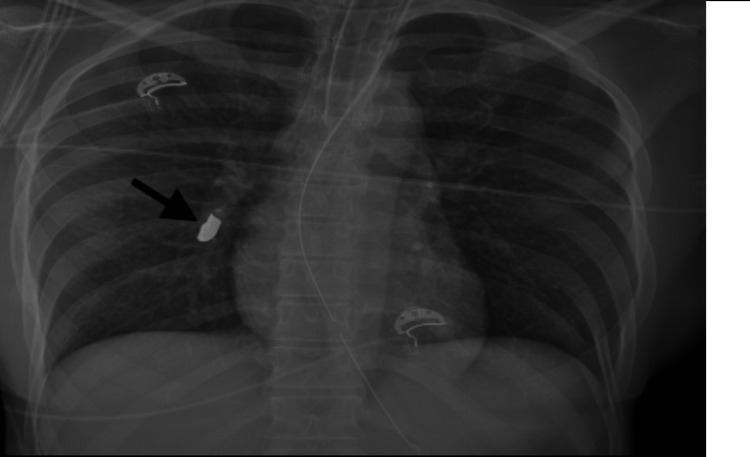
AP chest radiograph: Ballistic fragment projecting over the right lower lung zone. No subcutaneous emphysema or pneumothorax visualized to suggest direct penetrating trauma to the thorax. AP: anterior posterior.
